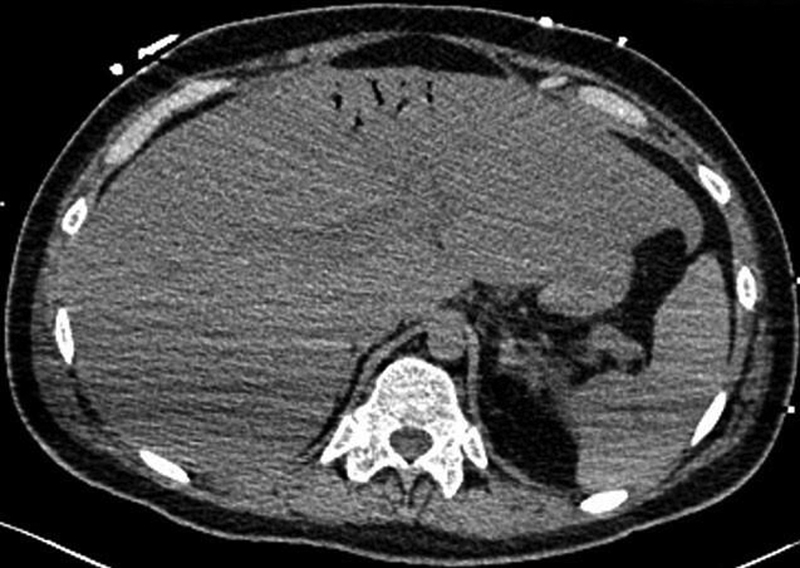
Axial noncontrast computed tomography (CT) scan image showing hepatic portal venous gas in the left portal vein branches.
